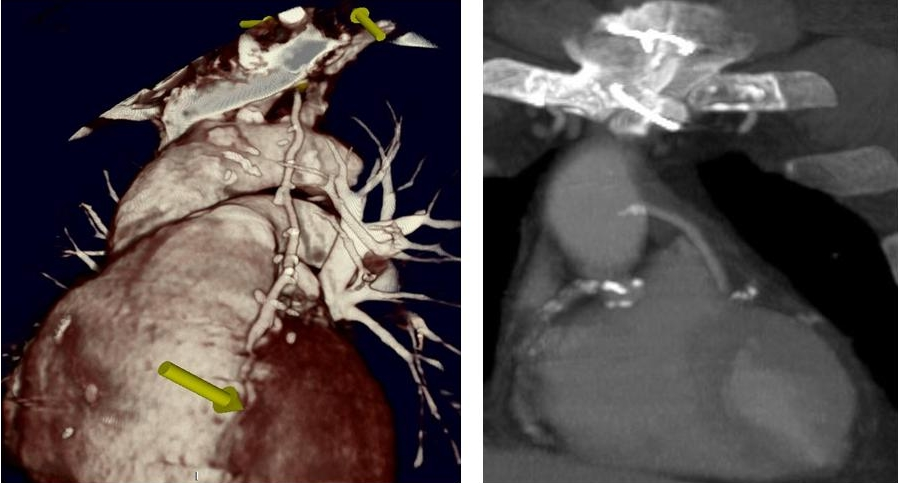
Postoperative Reconstruction CT Coronary Angiography (LIMA on LAD and SVG on first Diagonal).
